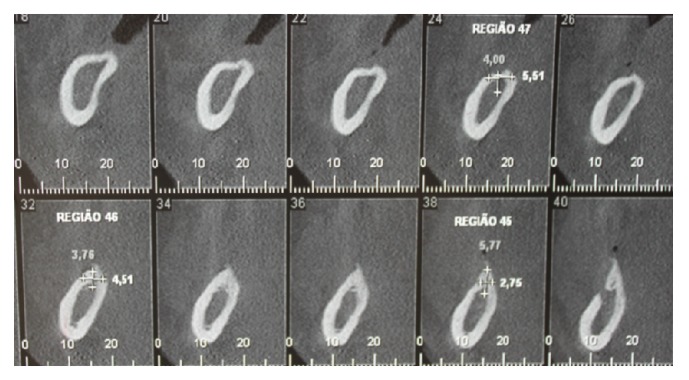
Computed tomography (cone beam) of the right mandible.
